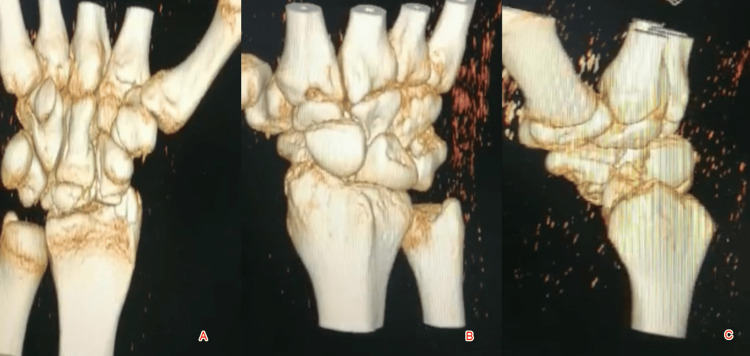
A CT scan of the right wrist with 3D reconstruction showing comminution of the distal scaphoid and the volar part of the lunate and displaced fracture of the tip of the radial styloid A: Anteroposterior view; B: Oblique/Scaphoid view; C: Lateral view
